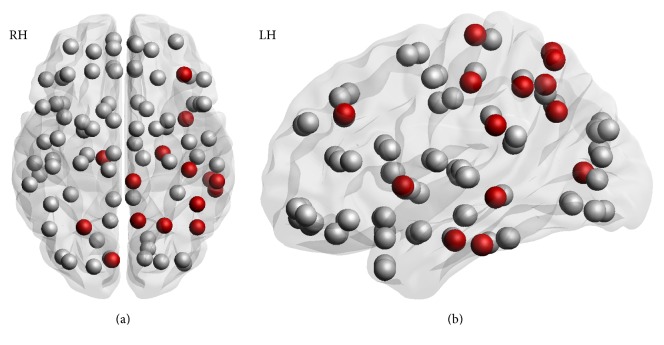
Axial (a) and lateral (b) view of the disconnectivity profile of the patient (obtained from analyzing the DTI-MRI data). Nodes represent 90 cortical and subcortical brain regions [38]. The red nodes indicate regions with more than 1.5 standard deviations of decreased connectivity strength compared to 25 age-matched healthy controls. Note that nodes surrounding the ischemic zone are affected. In addition, however, nodes in the contralesional hemisphere and a frontal node are affected. This suggests that the stroke affected a network of brain regions.
